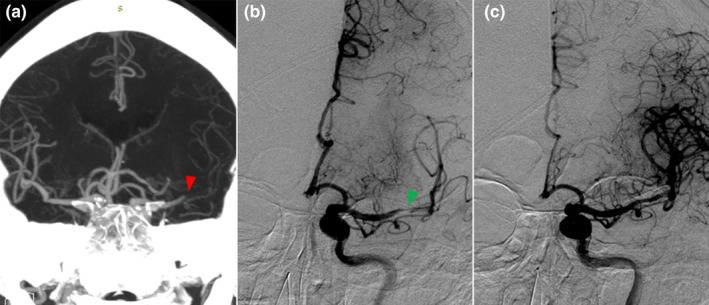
An example of early partial recanalization. (a) Admission computed tomographic angiography indicated complete occlusion of the M1 segment of the left middle cerebral artery (red arrowhead). (b) Tirofiban was administrated before endovascular thrombectomy. The modified Thrombolysis in Cerebral Infarction (mTICI) grade was 2a on initial angiography, indicating early partial recanalization (green arrowhead). (c) After one‐time mechanical thrombectomy and balloon dilation, successful recanalization was achieved, with an mTICI grade of 3 at the final angiography.
